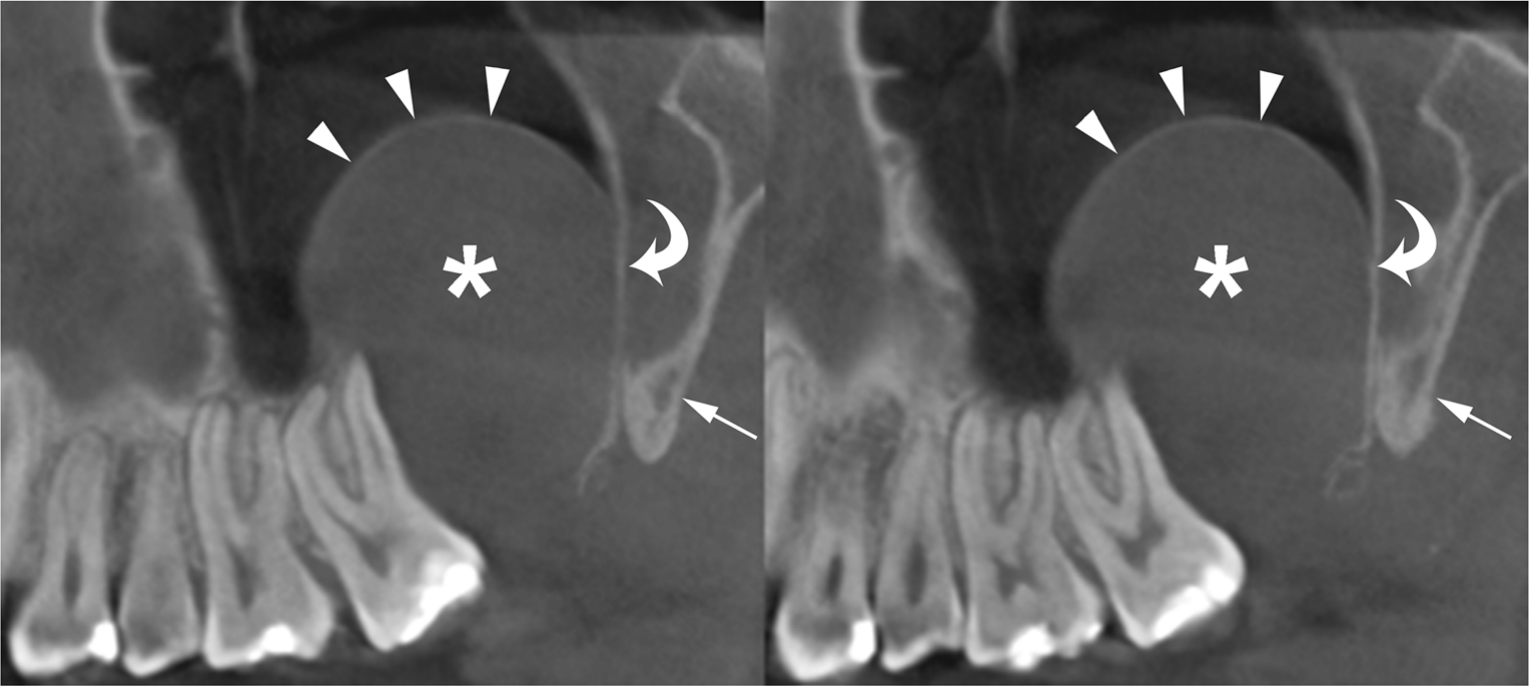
Panoramic CBCT images of a maxillary OKC (*asterisks*) originating from the molar region distally to the second molar tooth. The OKC causes significant sinus floor elevation (*arrowheads*). *Curved arrows*, posterior wall of the maxillary sinus; *arrows*, lateral pterygoid lamina
